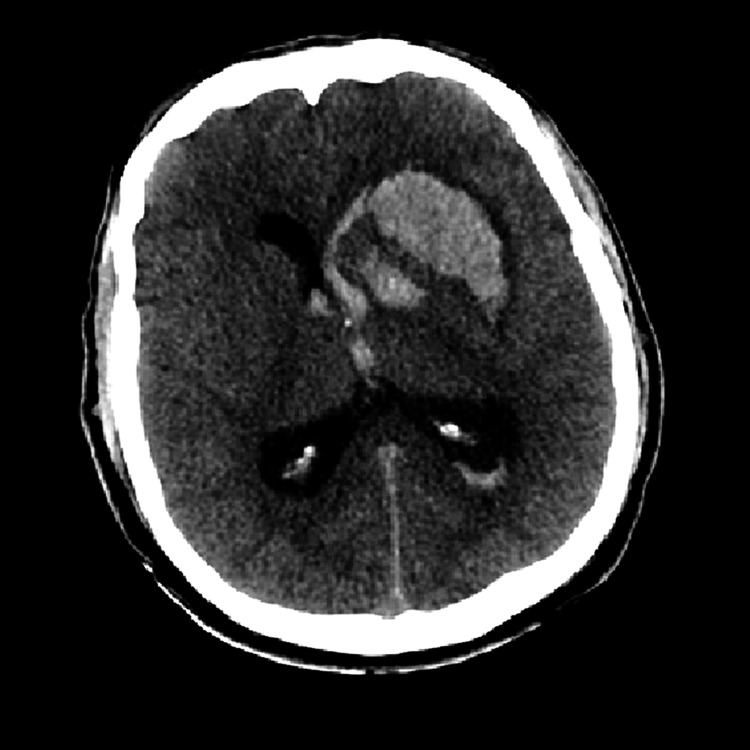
CT of the brain showing intraventricular hemorrhage CT: computed tomography
